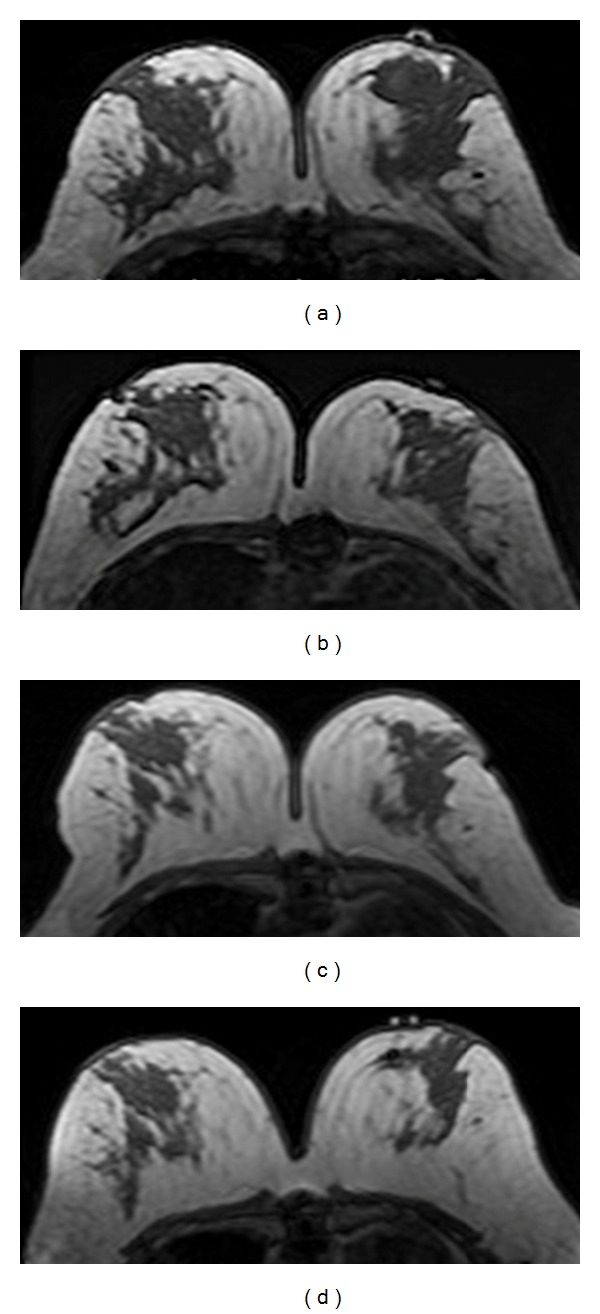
This is the same 41-year-old patient shown in Figure 3. The cancer is in the left breast, and the breast density is measured from the normal breast in the right side. From (a) to (d), the non-fat-sat T1-weighted images selected from the same level in pretreatment, F/U-1, F/U-2, and F/U-3 MRI are shown. It is noticed that the normal breast density decreases with chemotherapy. The measured percent density (fibroglandular tissue volume divided by the breast volume) is 13.4% before treatment, which decreases to 9.2% in F/U-1 after receiving 2 cycles of AC regimen and further down to 8.7% in F/U-2 and 8.2% in F/U-3.
